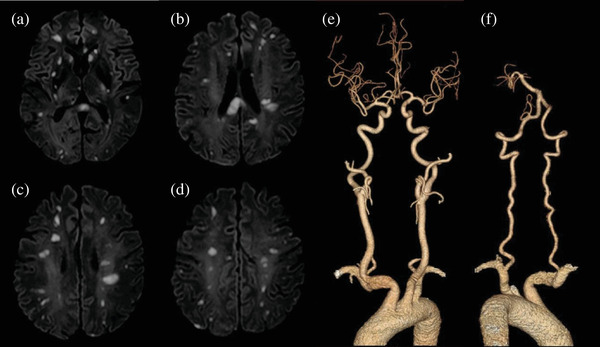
Typical DWI and CTA in a patient with HCRCI. A 51‐year‐old patient without cerebrovascular abnormalities and without vascular risk factors experienced acute cerebral infarction while hospitalized for treatment of hepatocellular carcinoma. DWI, picture A, B, C, and D, shows multiple high‐signal lesions in multiple arterial regions of the brain. CTA, picture E and F, shows bilateral carotid and vertebral artery systems are normal. DWI, diffusion‐weighted imaging; CTA, computed tomography angiography.
